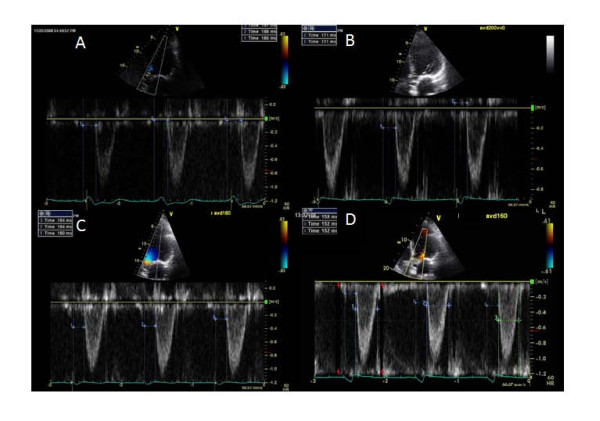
Effect of Biventricular Pacemaker Optimization on PEP Measured by Echo Doppler. Echo determined PEP in the same patient as in Figure 1 during native AV conduction at an AVD of 300 ms in panel A, baseline AVD of 200 ms in panel B, AVD of 180 ms in panel C and optimal AVD of 160 ms in panel C. Mean PEP was 184 ms in A and 171 ms at baseline AVD of 200 ms during biv pacing in B. AVD of 180 ms in C and optimal AVD of 160 ms in D led to shortening of PEP to 154 ms. Note difficulty in determining QRS onset in panel A.
